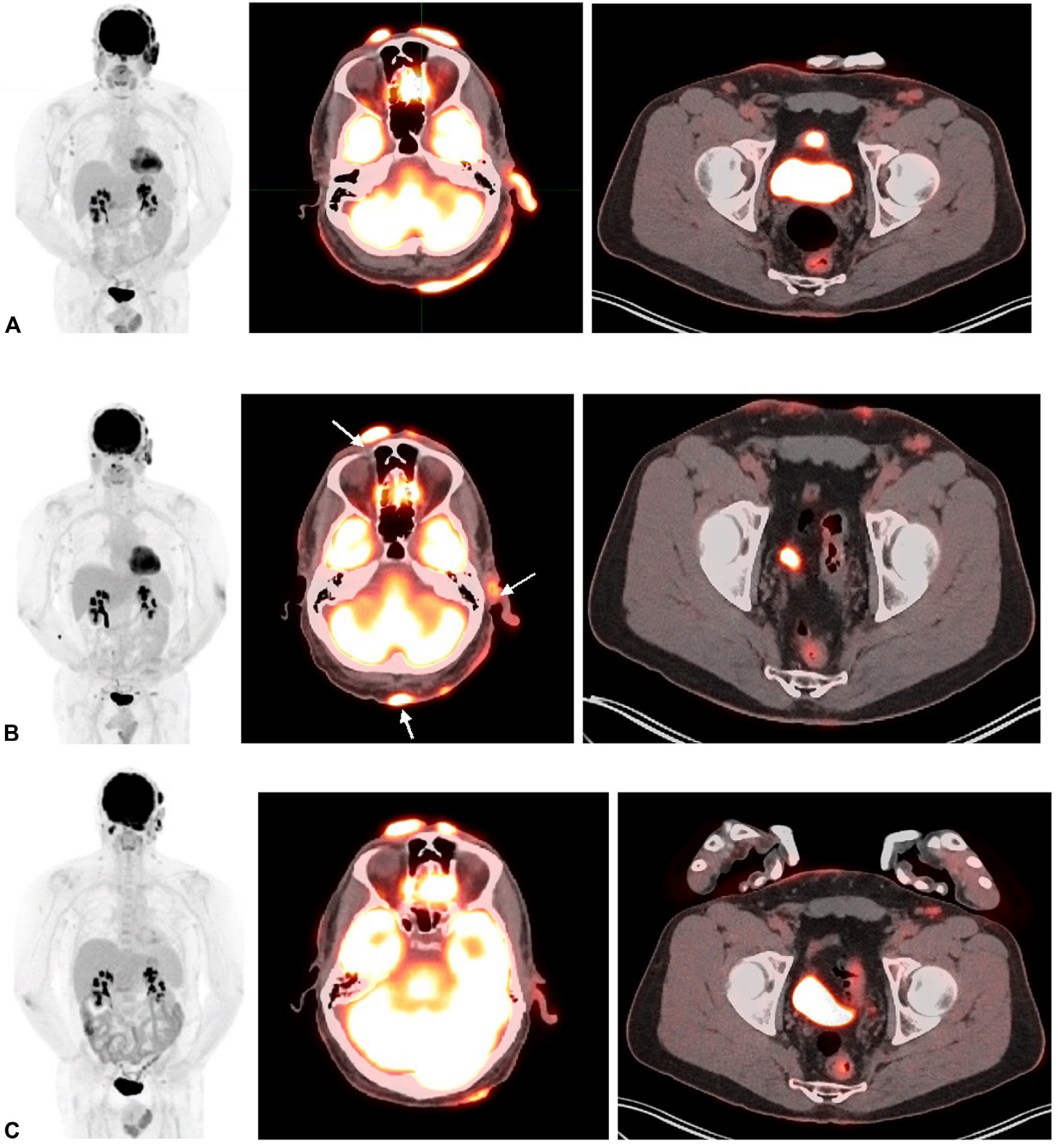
^18^F-FDG PET/CT results pretreatment (A) and following 2 (B) and 4 cycles of treatment (C). A, Numerous hypermetabolic cutaneous lesions throughout the scalp, most prominent on the *left* with involvement of the *left* ear (SUV_max_ up to 16 in the occipital region, *white arrows*, *middle* panel). Mild cutaneous thickening with mild FDG uptake in lower anterior abdominal wall is noted (*white arrow*, *right* panel). Mildly hypermetabolic lymphadenopathy in the bilateral neck, axillary, and inguinal regions. B, Significant interval decreases in size and FDG uptake of the scalp lesions, with some lesions completely resolved. Some lesions show interval worsening (ie, *right* nasal ala lesion, SUV_max_ 8.2, previously 3; *left* preauricular lesion, SUV_max_ 8, previously 3.3, *white arrows*, *middle* panel). There is slight interval increase in size of hypermetabolic skin thickening and new subcutaneous soft tissue nodules in the lower abdominal region. There is grossly stable mildly hypermetabolic lymphadenopathy in the bilateral neck, axillary, and inguinal regions. C, Mixed interval changes regarding the multifocal cutaneous scalp hypermetabolic lesions as some are improved while others are worsened. Improved anterior lower abdominal wall cutaneous hypermetabolic lesions. Grossly stable mildly hypermetabolic bilateral cervical lymph nodes. Slightly less conspicuous FDG uptake in bilateral axillary and inguinal lymph nodes. *^18^F-FDG PET/CT*, 18F-fluorodeoxyglucose positron emission tomography-computed tomography; *SUV*_*max*_, maximum standardized uptake value.
